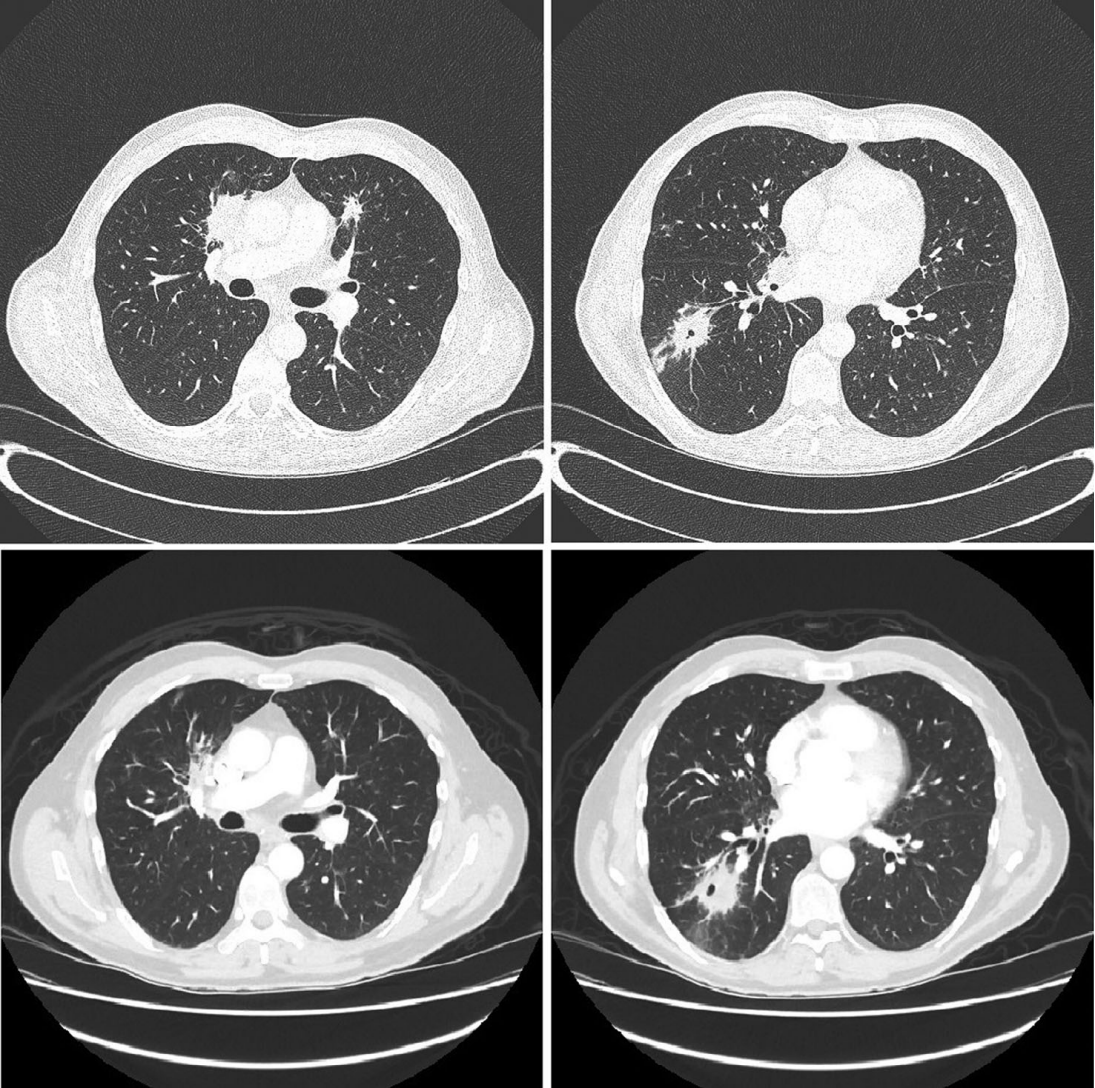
Lung high‐resolution computed tomography (HRCT). Multifocal consolidation and right lower lobe cavitary lung mass on presentation (top two panels) and after 10 weeks of prednisone (lower panels).
